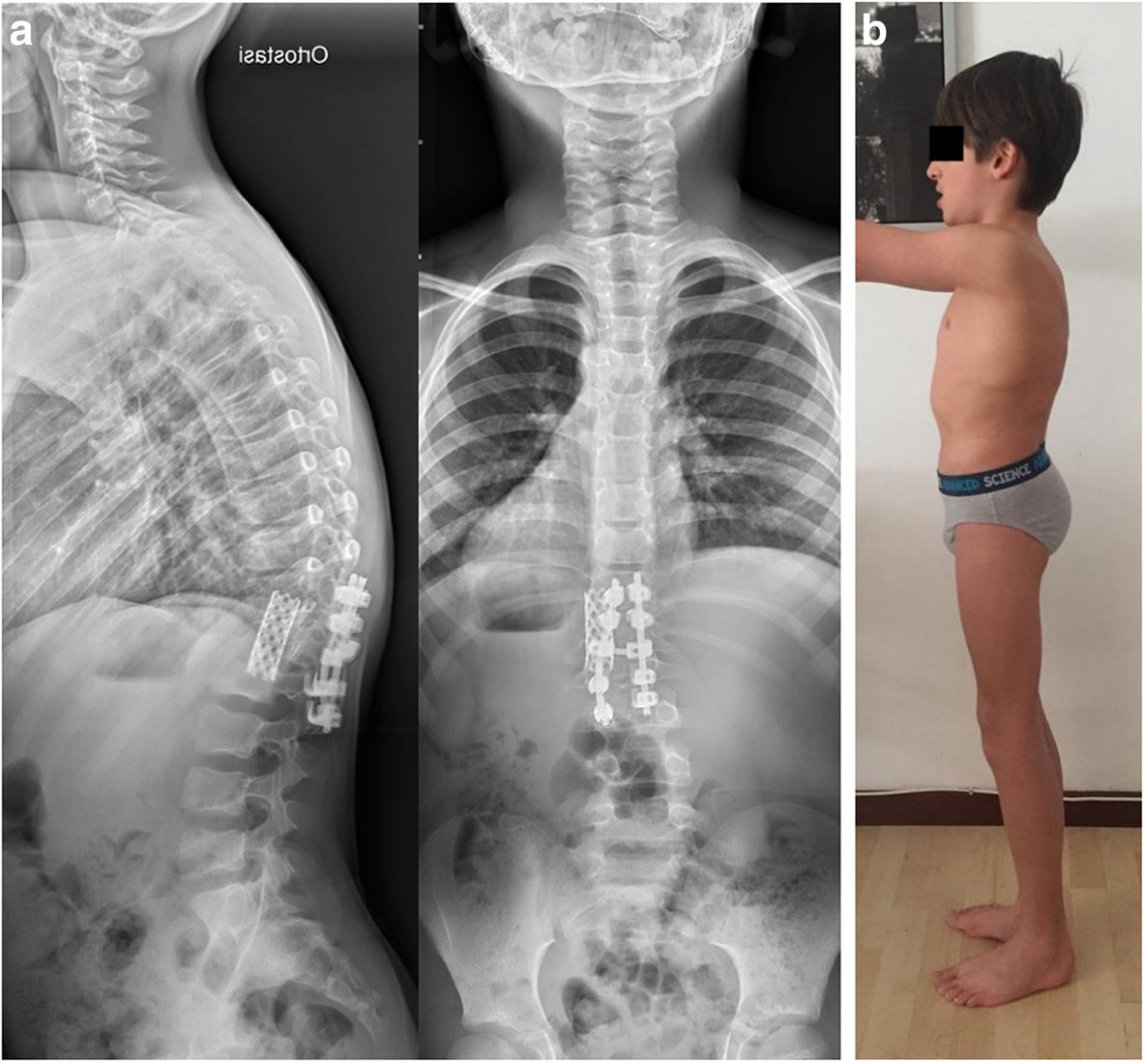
Standing radiographs (a) and clinical aspect (b) showing the permanent deformity correction at 9 years of age
